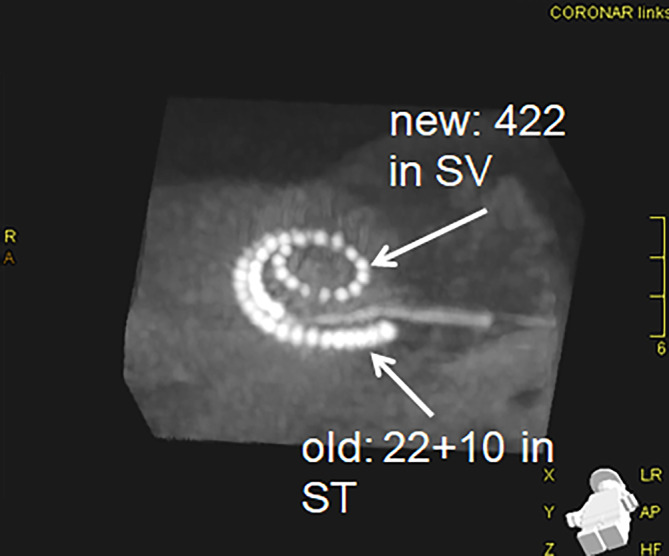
Cone-beam computed tomography image of the petrous bone with original electrode array (*ET*) in the scala tympani (old ET: 22 + 10 Cochlear^TM^) and subsequently inserted electrode array in the scala vestibuli (new ET: 422 Cochlear^TM^)
